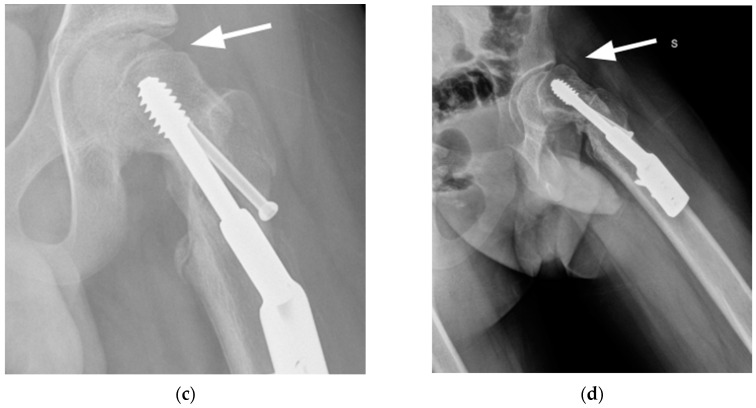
(a) Radiograph after trauma showing a Delbet Type III femoral neck fracture; (b) radiograph after open reduction and internal fixation surgery; (c) radiograph at one-month follow-up showing signs of mild SCFE (white arrow); (d) radiograph at three-month follow-up showing worsening SCFE (white arrow).
